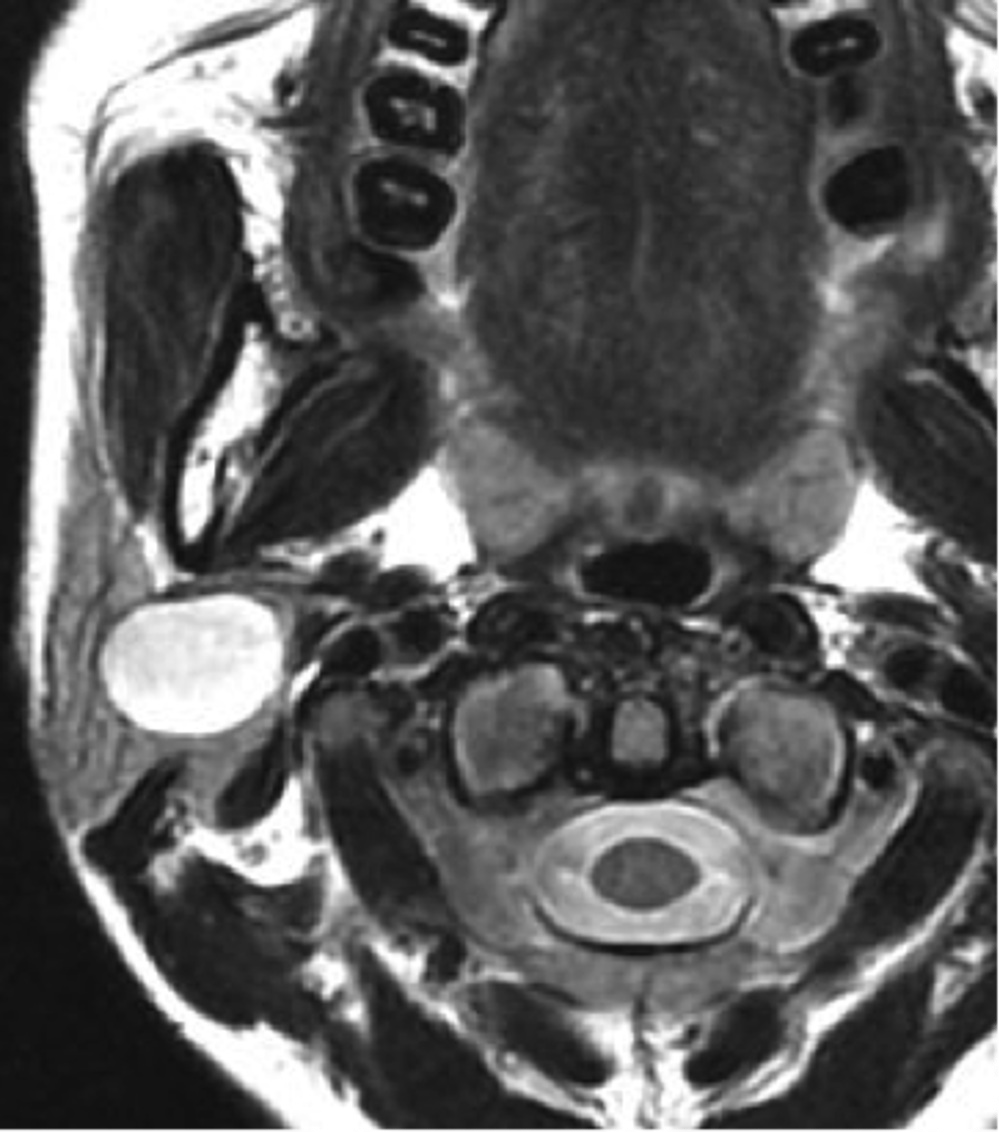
Axial T2-weighted image showing a heterogeneous, hyper-intense, cystic lesion in the right parotid.
